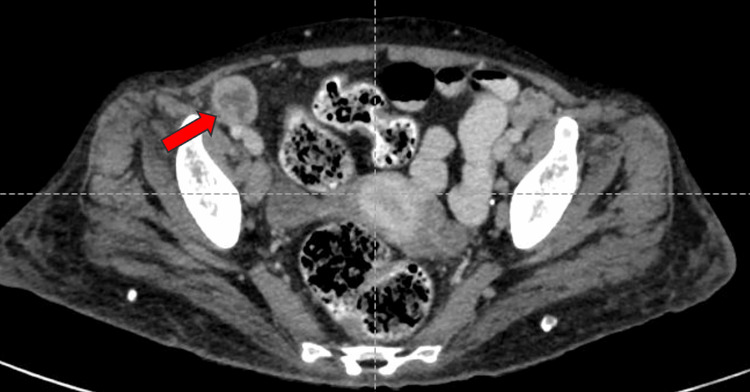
CECT-AP axial section showing an enlarged, non-enhancing necrotic lymph node along the right iliac vessels (right obturator node) shown by the red arrow. CECT-AP: contrast-enhanced computed tomography of the abdomen and pelvis
